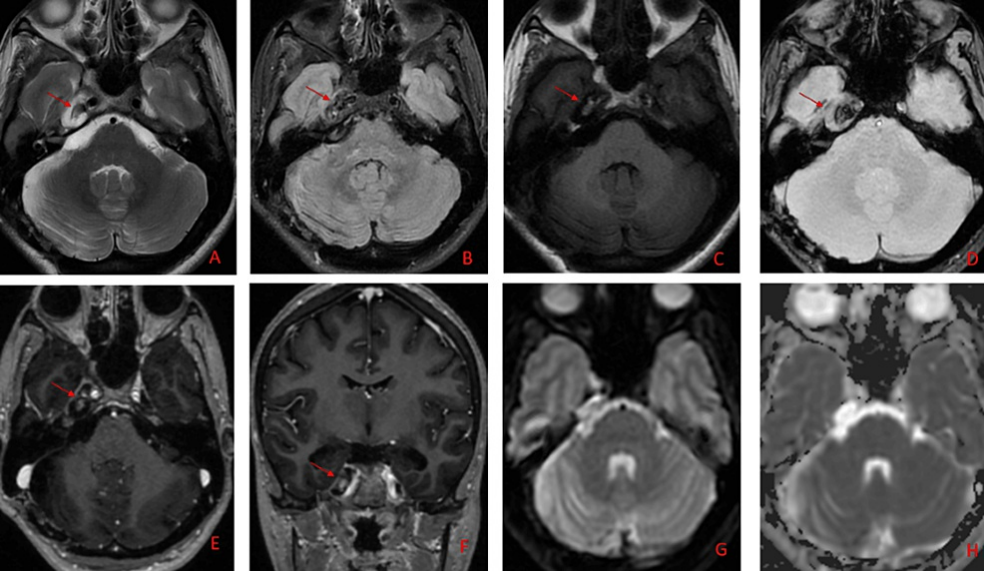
Post-operative MRI brain showed a residual lesion in the right Meckel's cave having hypointense signals to gray matter on T2 (image A) and FLAIR (image B), gradient imaging (D), and hyperintense signals on T1 WI (C). It abuts the right seventh and eighth nerve complex and a cavernous segment of the internal carotid artery. Minimal enhancement on axial (E) and coronal (F) post-I/V Gadolinium contrast images was also observed. No restriction on DWI (G) and ADC (H) images DWI: Diffusion-weighted imaging; ADC: Apparent diffusion coefficient; FLAIR: Fluid-attenuated inversion recovery; WI: Weighted imaging
